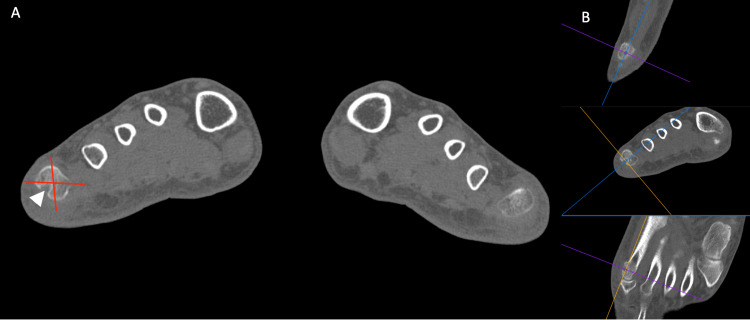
Computed tomography of both forefeet with multi-planar reconstructions. (A) Bone erosion (white arrowhead) located at the fibular quadrant (FQ) of the fifth metatarsal head of the right foot (image acquired at 2 mm of thickness and reconstructed at 2 mm of thickness; (B) the multi-planar reconstruction (MPR) centered on the focal bone loss confirms the presence of the bone erosion. The red lines represent the division in quadrants.
